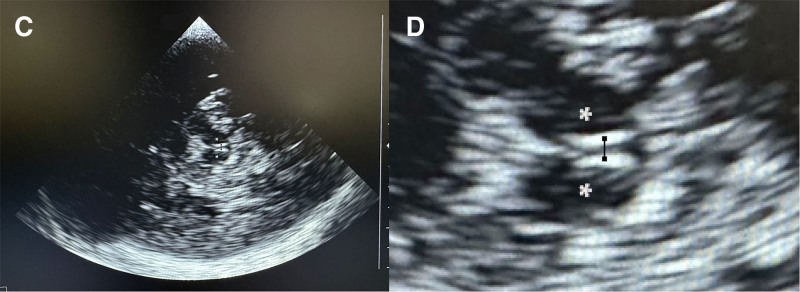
Transcranial ultrasound assessment of the third ventricle in the same patient. A, Insonation through the temporal acoustic window demonstrating the hyperechogenic margins of the third ventricle, surrounded by the hypoechogenic thalami (*asterisks*). B, Zoomed view of the third ventricle highlighting precise caliper placement between the echogenic ventricular walls.
